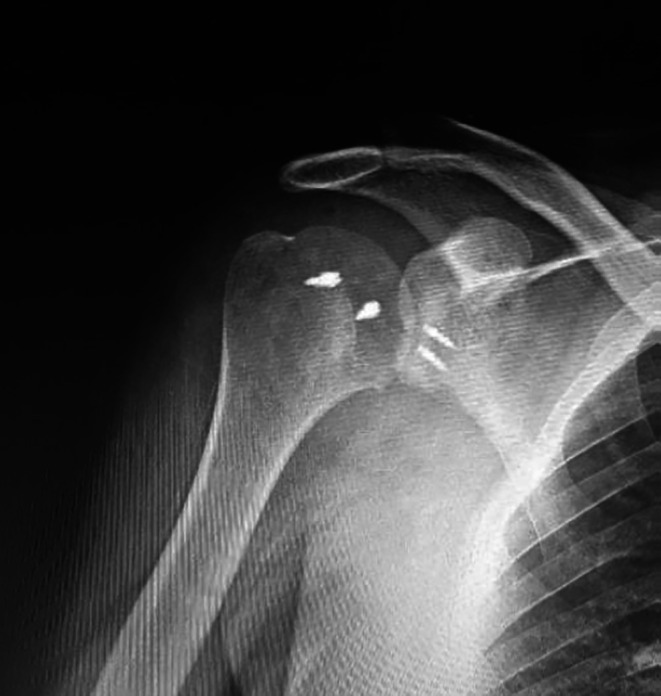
Postoperative radiograph of right shoulder after closed reduction and arthroscopic Mclaughlin procedure with posterior labral repair.
